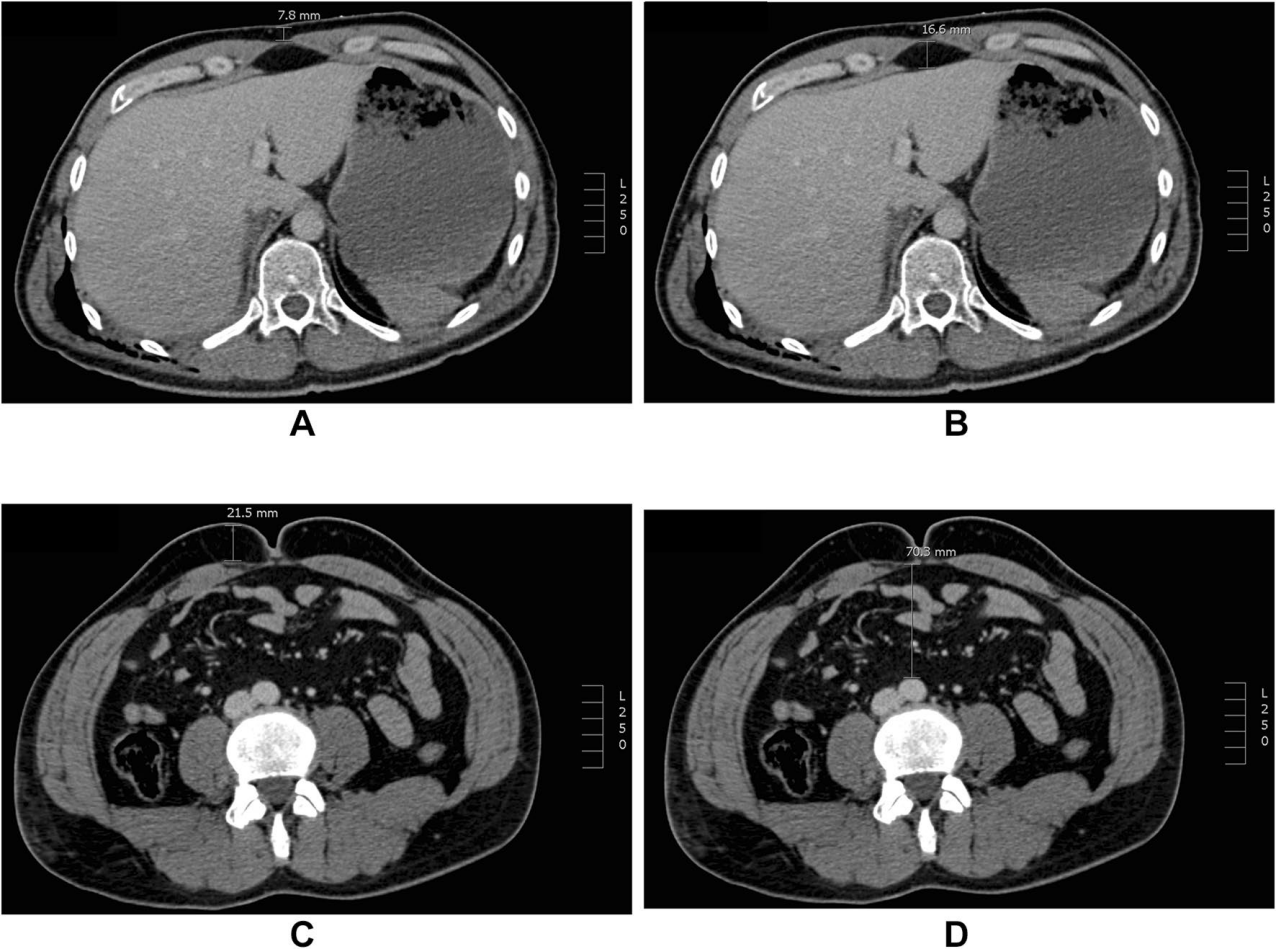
Computed tomography (CT)-based measurements. a Subxiphoid outer abdominal fat (SOAF) on an axial plane of a CT scan. b Subxiphoid peritoneal fat (SPF) on an axial plane of a CT scan. c Umbilical outer abdominal fat (UOAF) on an axial plane of a CT scan. d Umbilical visceral fat (UVF) on an axial plane of a CT scan
